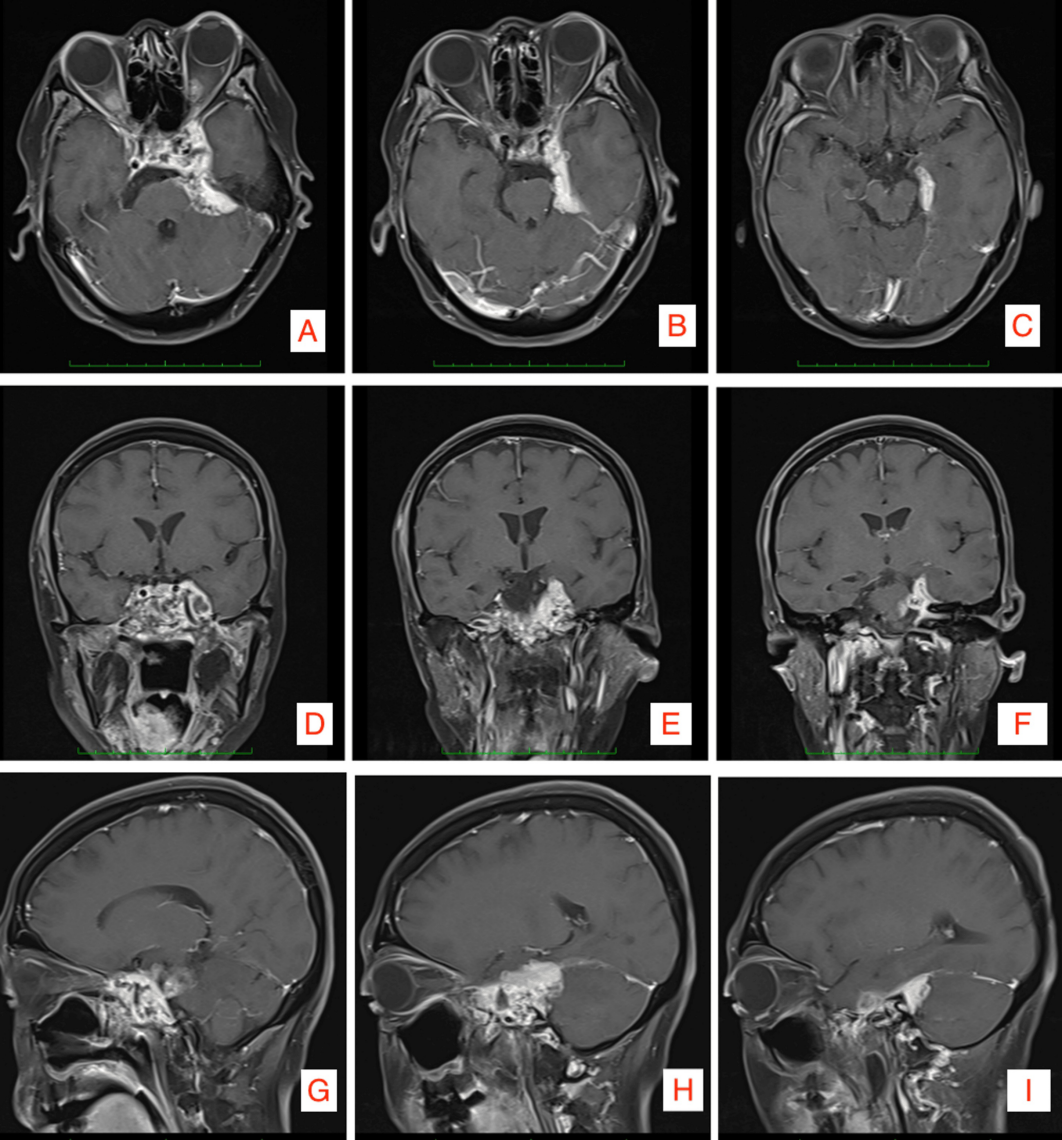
Preoperative contrast MRI. A-C: (axial view) Noted enhancing mass invading the bilateral cavernous sinus, the left antero-lateral pons, and left medial temporal lobe. D-F: (coronal view) Tumor invasion enveloping the left and right (not shown) cavernous segment of the internal carotid artery, left infero-lateral pons and uncus. G-I: (sagittal view) nasopharyngeal tumor extension to the clivus and pre-pontine cistern.
